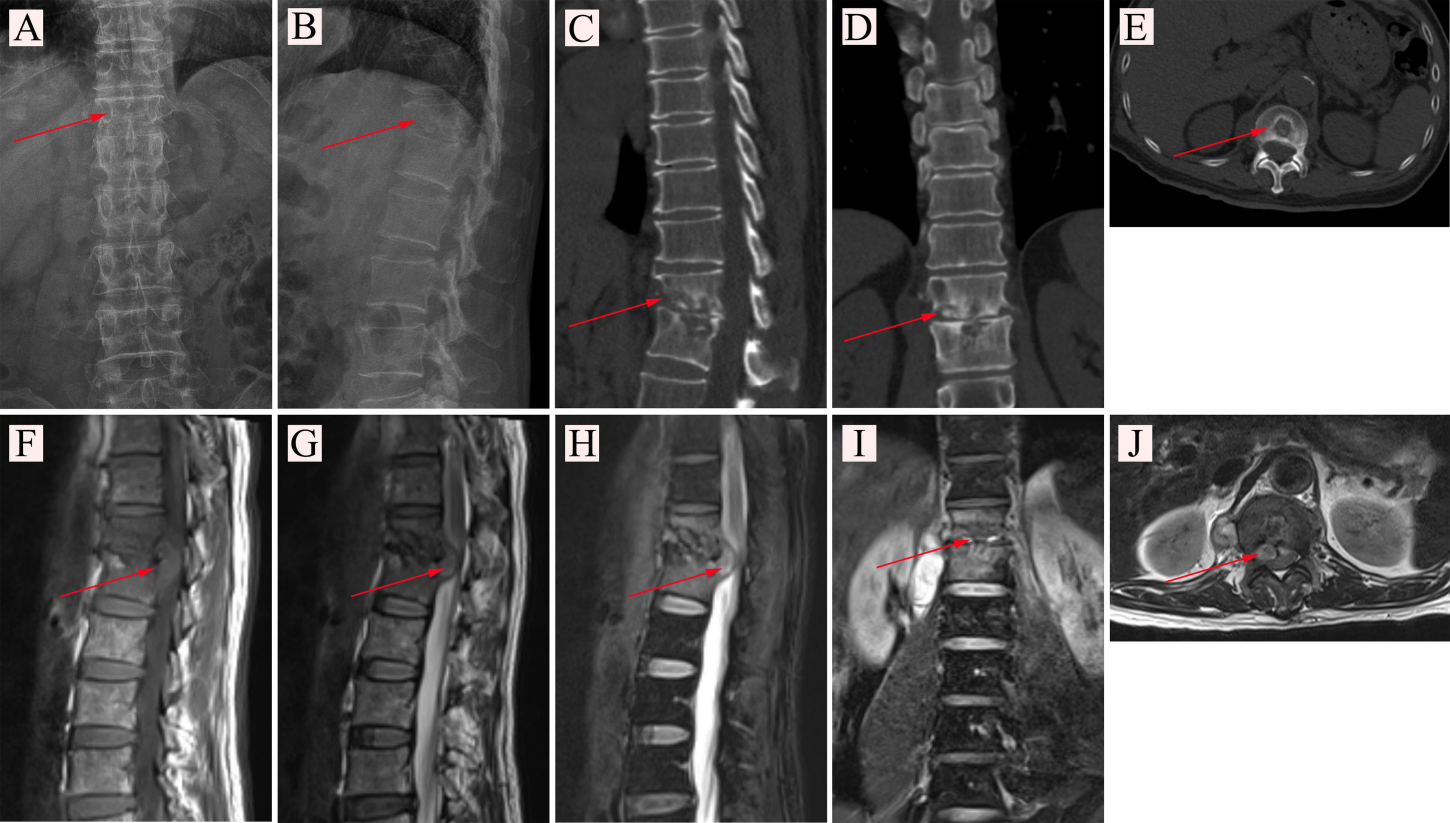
(A, B) X-ray examination of a patient with spinal tuberculosis. The arrow points to the location of the lesion. (A) X-ray image in posterior-anterior position. (B) X-ray image in lateral position. (C–E) CT examination of a patient with spinal tuberculosis. The arrow points to the location of the lesion. (C) CT image in sagittal position. (D) CT image in coronal position. (E) CT image in cross-section. (F–J) MRI examination of a patient with spinal tuberculosis. The arrow points to the location of the abscess. (F) MRI image in the sagittal T1 sequence. (G) MRI image in the sagittal T2 sequence. (H) MRI image of T2 lipid compression sequence in sagittal position. (I) MRI image in coronal position. (J) MRI image in cross-section.
